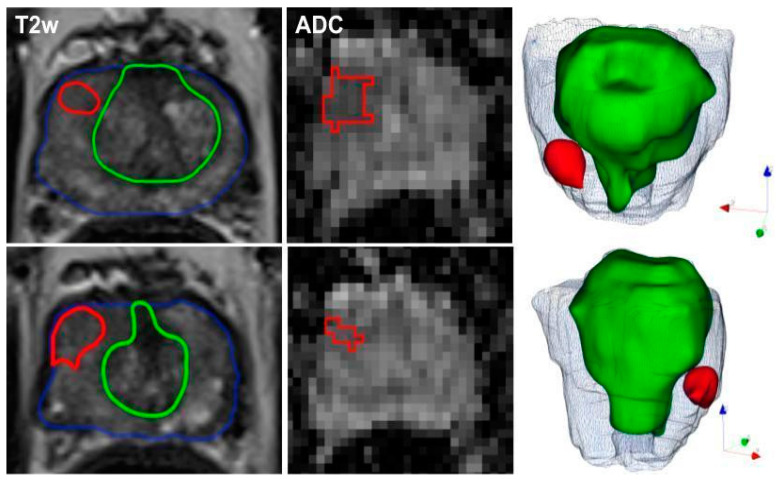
Representative segmentation of a 60-year-old man on axial T2-weighted and ADC-images with an initial prostate-specific antigen level of 12.9 ng/ml, normal DRE result, and a highly suspicious lesion located medio-apically in the right peripheral zone (PI-RADS category 4). Targeted MRI/ultrasound-fusion biopsy confirmed the presence of PCa, Gleason 3+4, ISUP 2. The contours outline the following manual segmentations: blue—whole organ, green—transition zone, red—index lesion.
